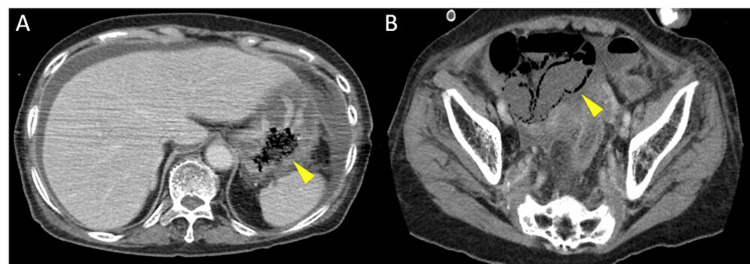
Contrast-enhanced abdominal computed tomography Contrast-enhanced CT images show areas of contrast defects suggestive of ischemia or necrosis (arrowheads). A: gastric fundus and the upper body of the stomach; B: small intestine
